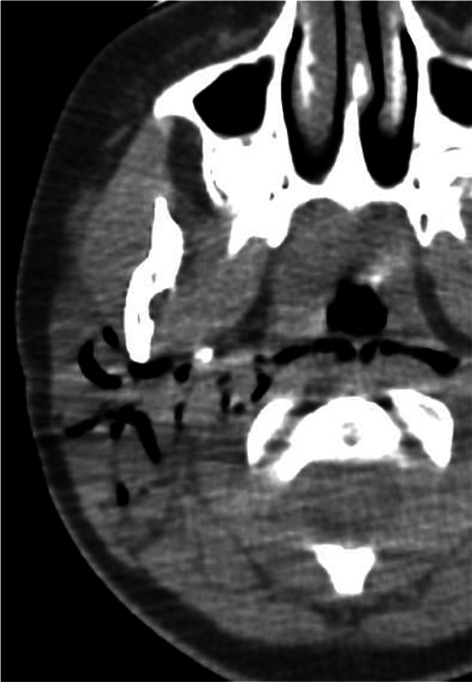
Right pneumoparotid complicated with deep cervical emphysema, of unknown etiology, on axial CT image. *Courtesy Dr. M. Mabille (CHI Créteil, France)*
